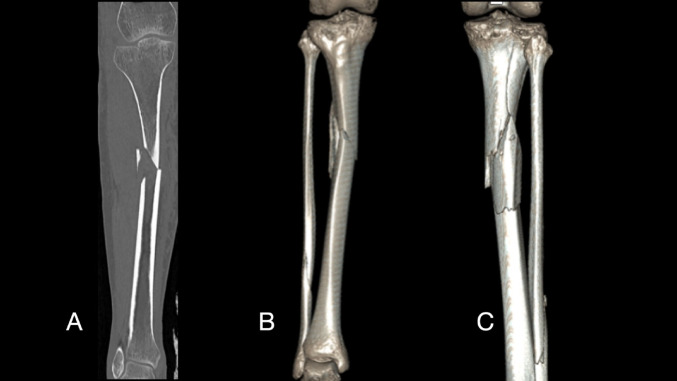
Coronal computed tomography (CT) scan (A) and three-dimensional reconstructions (B: anterior view; C: posterior view) of the leg. Note the intra-articular extension and the presence of a Meyers and McKeever type I tibial spine fracture, as modified by Zaricznyj [1]
